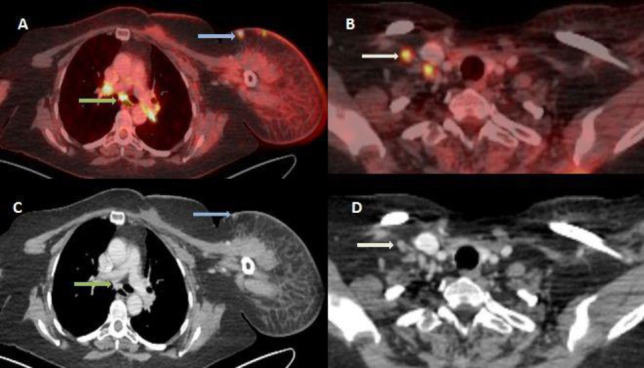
Fused axial FDG PET/CT (A and B) and corresponding CECT image (C and D) show FDG avid bilateral hilar and subcarinal (SUV_max_ 8.8) lymph nodes (yellow arrow), right supraclavicular lymph nodes (SUV_max_ 7.4; white arrow) and few subcentimetric subcutaneous nodules (SUV_max_ 5.1; light blue arrow).
